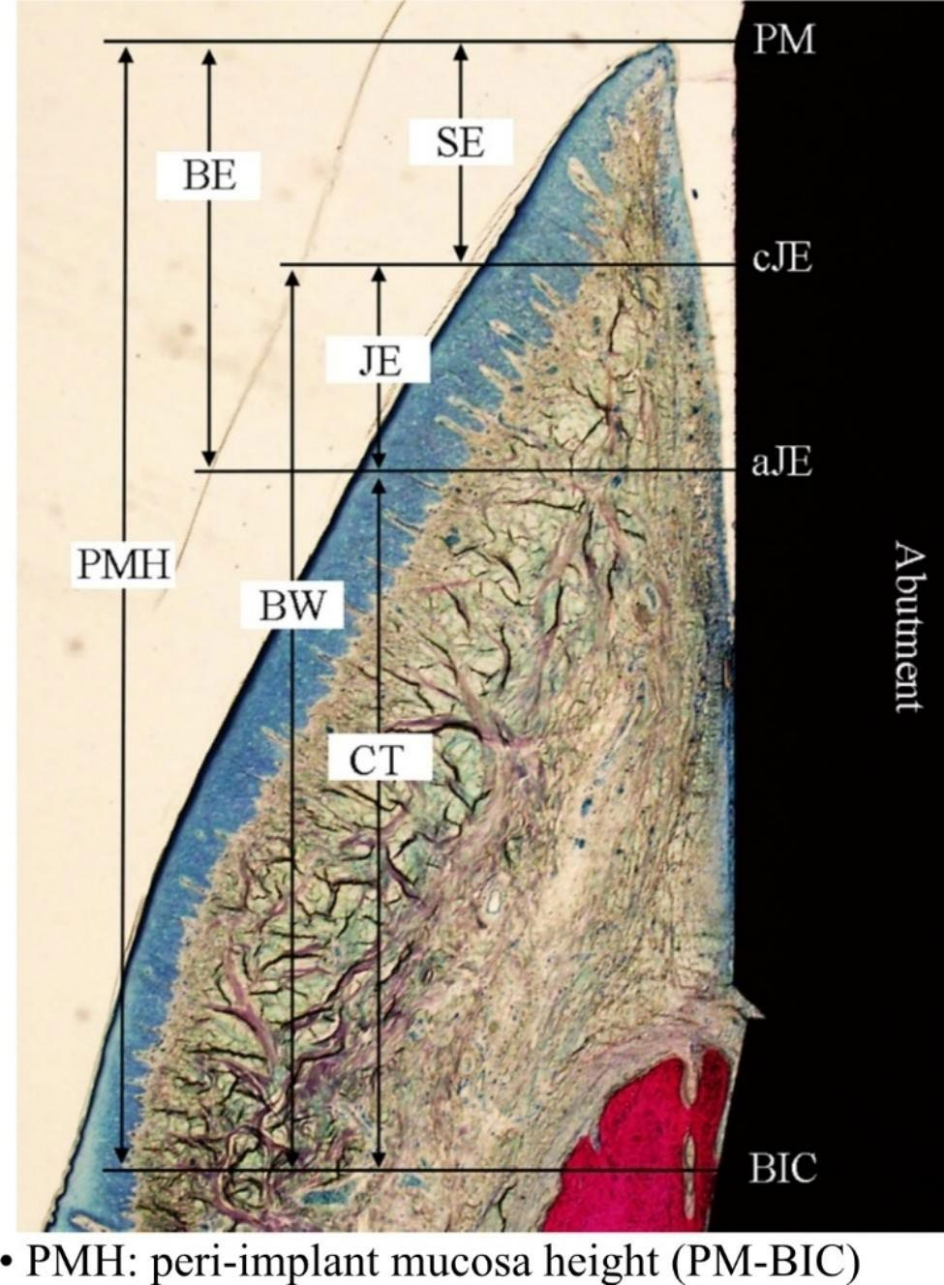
Landmarks for histometric evaluation. PM, peri-implant mucosa margin; cJE, most coronal point of the junctional epithelium; aJE, apical extension of the junctional epithelium; BIC, most coronal portion of the bone-implant contact. Linear measurements between landmarks: SE, length of sulcular epithelium (PM-cJE); JE, length of junctional epithelium (cJE-aJE); BE, barrier epithelium consisted of the dimension of SE and JE (PM-aJE); CT, length of connective tissue (aJE-BIC); BW, biological width consisted of the dimension of JE and CT (cJE-BIC); PMH, peri-implant mucosa height (PM-BIC).
